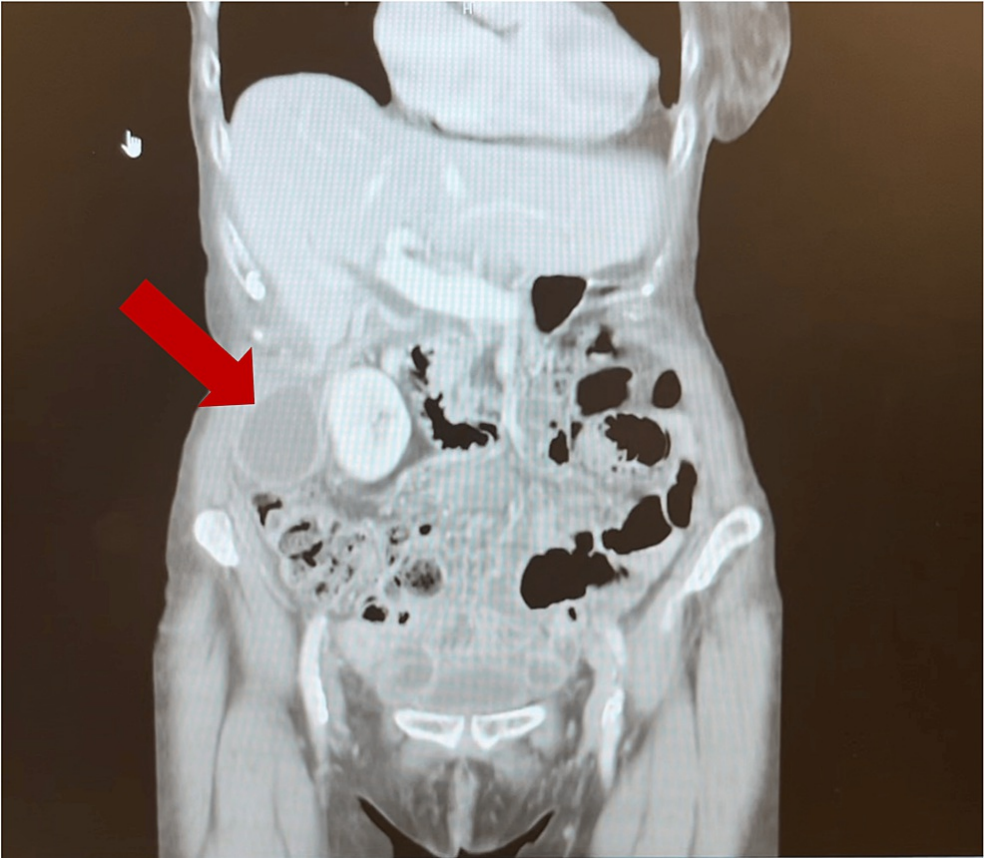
Alternative view of CT showing the gallbladder in the paracolic gutter/lateral to the right kidney
